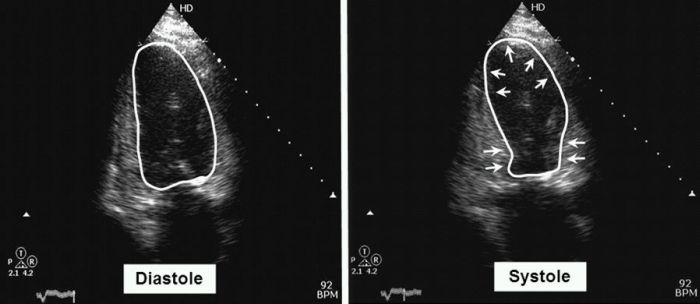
Echocardiogram clearly showing apical ballooning during systole with normal contraction of the basal segments (arrows)
